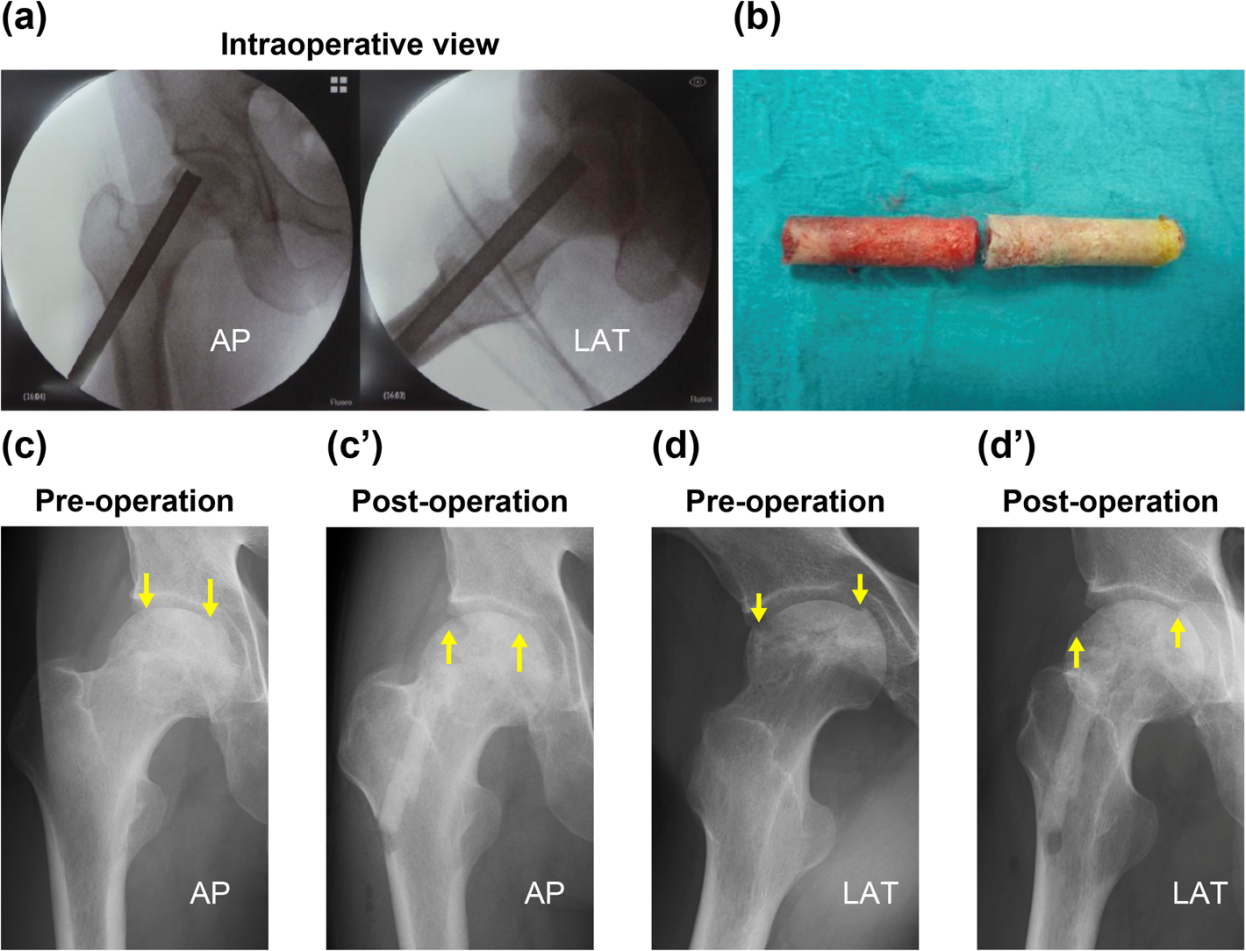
Surgical procedure, as shown in pre- and postoperative radiographs and intraoperative photographs. a Representative intraoperative fluoroscopic image at CD. b Photograph of a cylindrical bone taken by CD. The bloody zone is healthy, and the yellowish-white part on the right is the osteonecrotic part. Reverse IBG was performed. Postoperatively, the gap was repaired. Core defects in the healthy zone were supplemented with artificial bone avoiding fracture. c, c’ Changes on AP X-ray. d, d’ Changes on LAT X-ray. Downward yellow arrows indicate the gap made by femoral head collapse, while the upward yellow arrow indicates repair of the femoral head sphericity by IBG. CD, core decompression; IBG, impaction bone graft; AP, anteroposterior; LAT, lateral
